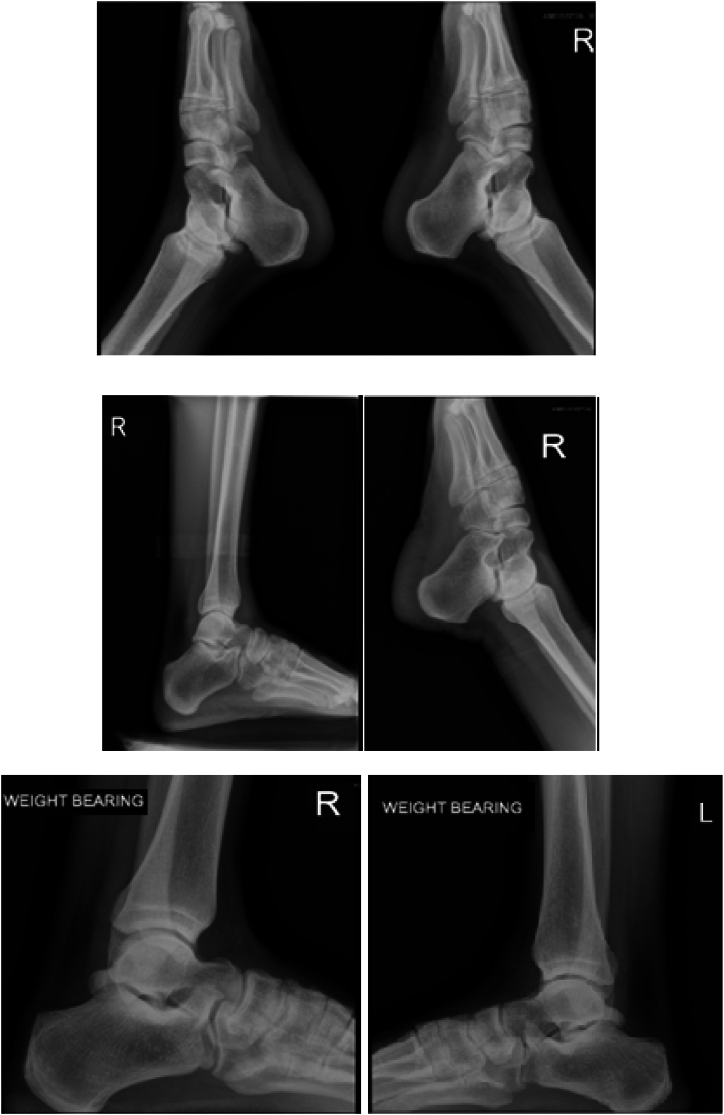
Series of X-ray images demonstrating various views of the ankle joints. Top row: Anteroposterior views of both ankles, showing normal bony alignment without signs of fracture or significant degenerative changes. Middle row: Lateral views of the right ankle in different flexion positions, highlighting the clear spaces and bone densities. Bottom row: Weight-bearing views of both the right (R) and left (L) ankles, useful for assessing alignment under physiological load conditions. These images were accessed for inclusion to rule out other bony abnormalities or other related pathologies.
